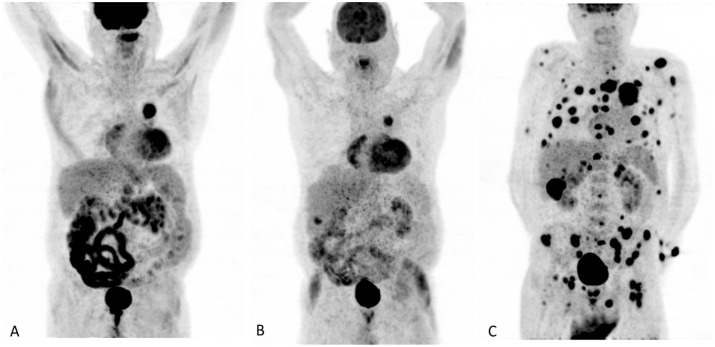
77-year-old man being followed because of an adenocarcinoma in a sigma polyp. He presented an incidental finding of a lung mass located in the superior left lobe, later confirmed as a lung adenocarcinoma with evidence of loco-regional lymphadenopathic infiltration. (A) Third [^18^F]FDG PET/CT showing radiological stability after chemotherapy. (B) Oligometastatic progression in liver parenchyma with solitary lesion after chemotherapy and radiotherapy. (C) After initiating immunotherapy (three cycles of Atezolimumab), [^18^F]FDG PET/CT evidenced progression of the primary tumour and the liver lesion with countless liver, adrenal and bone lytic lesions as well as peritoneal implants, consistent with hyperprogression. The patient died one month later.
